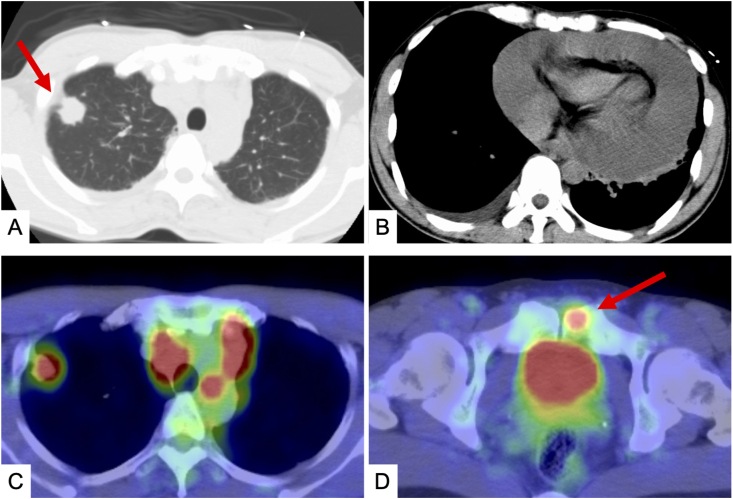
Imaging findings at the time of initial diagnosis A, B) Chest computed tomography scan shows a primary lesion in the right upper lobe and a massive pericardial effusion. C, D) F18 fluorodeoxyglucose positron emission tomography scan shows uptake by the primary lesion, mediastinal lymph nodes, and pelvic bone.
